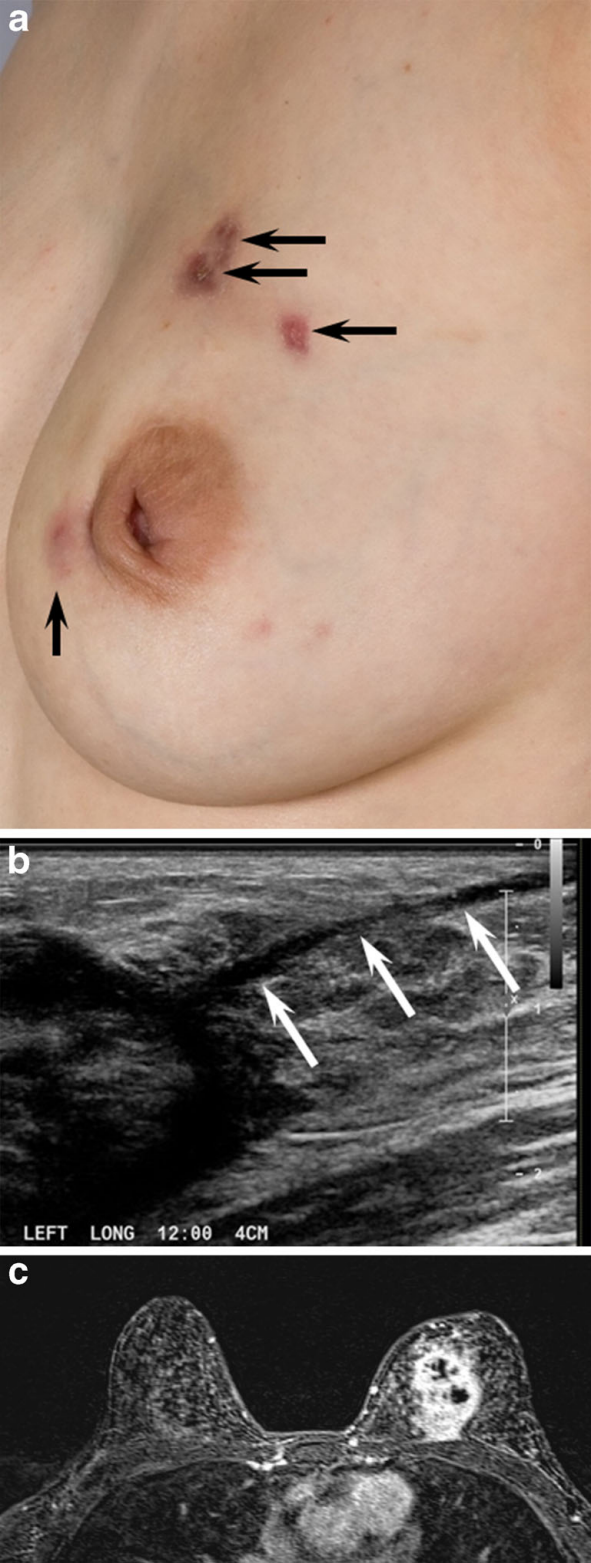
A 24-year-old Albanian mother of a 4-year-old (breastfed for 1 year) presented with a left breast lump, pain, erythema and drainage from multiple sites. Clinical photograph (a) of the left breast shows four eschars (*arrows*), two of which in the upper breast were draining cloudy fluid. Also shown is nipple inversion. b Ultrasound (US) image of the upper left breast shows a hypoechoic mass with indistinct margins with tract extending into adjacent tissue and to the skin surface (*arrows*) to the uppermost eschars noted the clinical photograph (a). c Axial T1-weighted post-contrast subtraction axial MRI image shows an irregular, intensely enhancing mass in the left breast extending to the chest wall with central areas of non-enhancement consistent with abscess formation. Ultrasound biopsy revealed IGM
